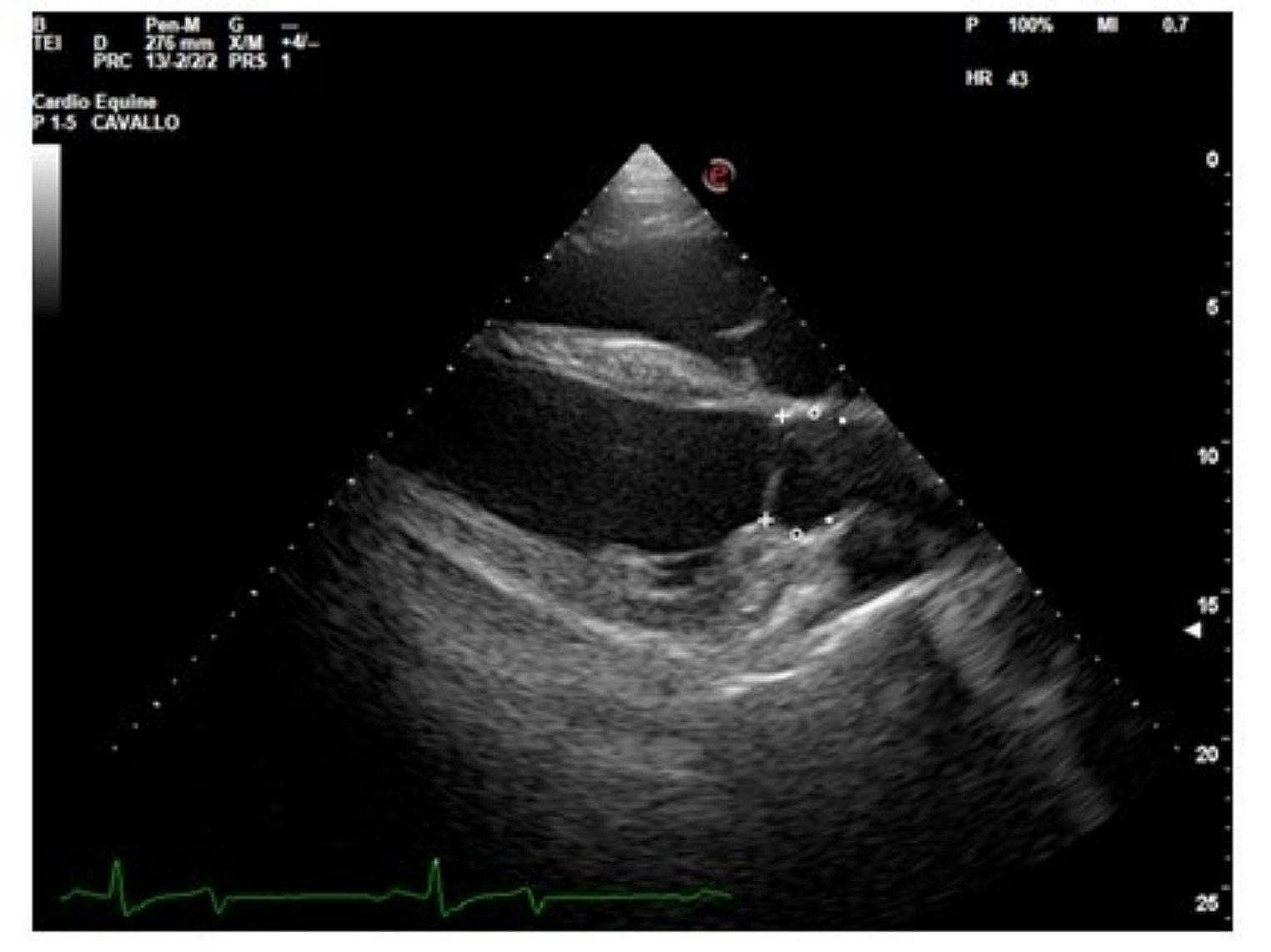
Right parasternal left ventricular outflow tract view showing the measurements of the aortic diameter at the base of the valve - ABS (+), the sinus of Valsalva level – ASV (°), and the sino-tubular junction - AJT (▪) at end-diastole
